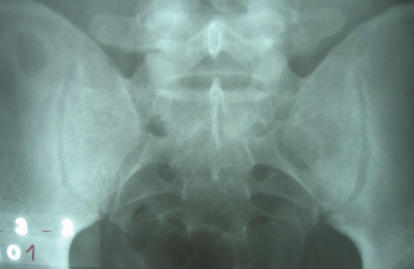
Plain Radiograph of the Sacroiliac Joints on Presentation to the Hospital San Juan de Matucana The radiologist reported this radiograph as normal.
